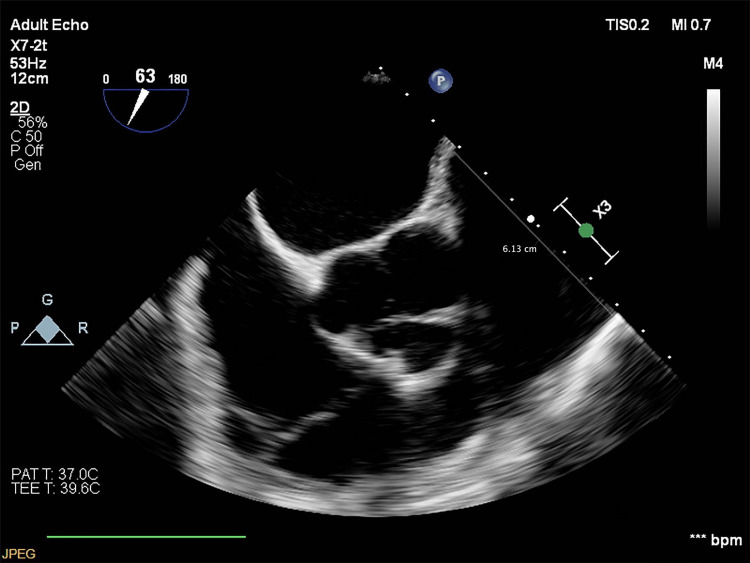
Perioperative transesophageal echocardiography from January 2023 Echocardiography indicates a pulmonary artery aneurysm measuring 6.1 cm.
